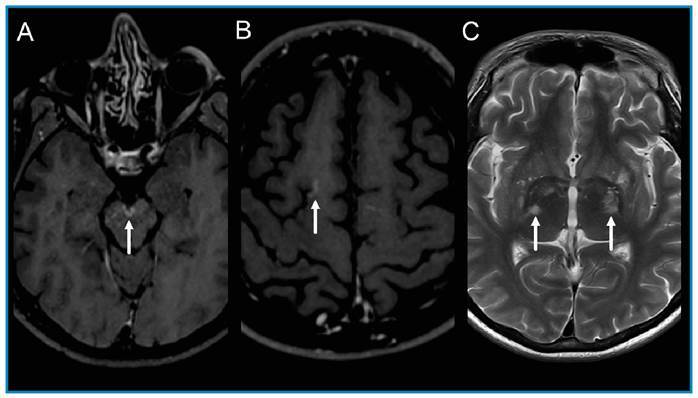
Post-contrast axial T1-weighted images showing leptomeningeal enhancement of the interpeduncular fossa and right superior frontal sulcus (arrows, A and B). Axial T2-weighted image showing dilated perivascular spaces in the basal ganglia (arrows, C).
